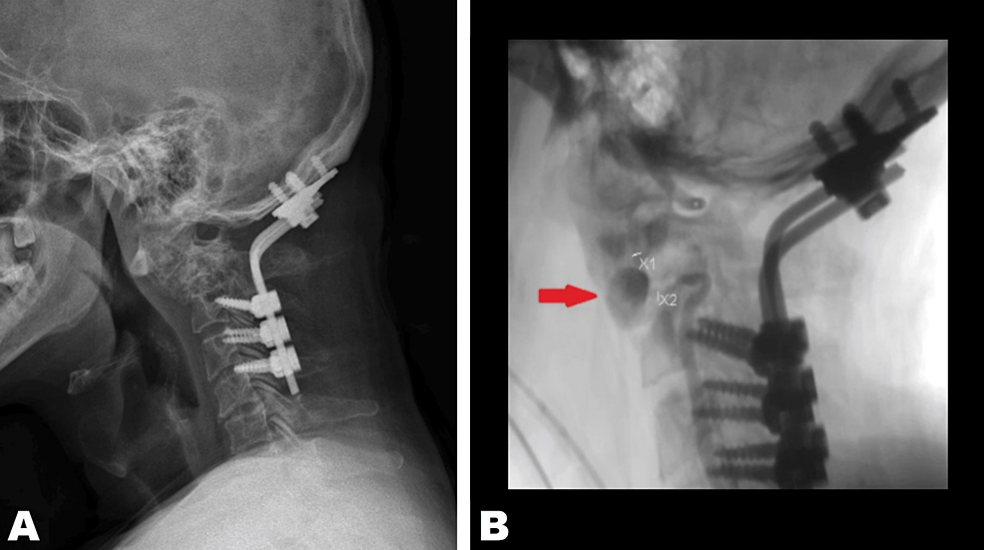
(A) Postoperative X-ray shows occipitocervical arthrodesis. (B) DSA shows a pseudoaneurysm in the V3 segment of the left vertebral artery (red arrow). DSA: digital subtraction angiography
